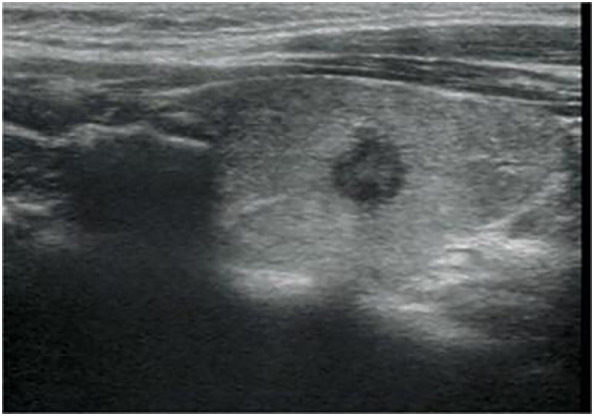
Longitudinal ultrasonography image from a 34-year-old woman with papillary thyroid carcinoma shows a 7.5-mm solid, hypoechoic, and irregular margin thyroid nodule with punctate echogenic foci, associated with no suspicious lymph node. The nodule was classified as EU-TIRADS 5:high risk or K-TIRADS 5:high suspicion. The nodular failed FNA based on nodule size.
